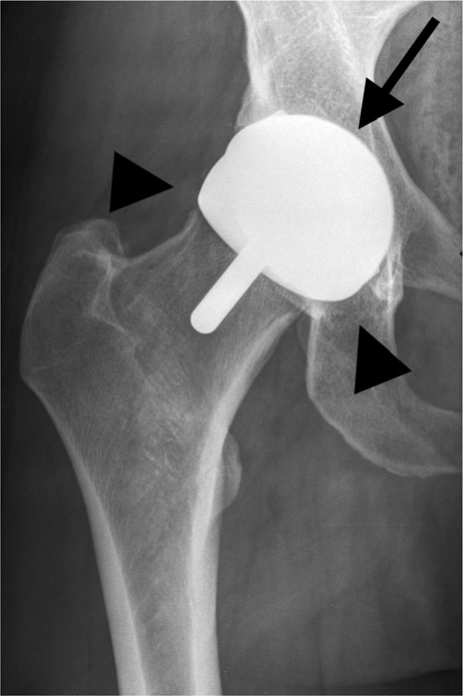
AP radiograph. Cementless resurfacing total hip arthroplasty. In a resurfacing total hip arthroplasty, the femoral head (*arrowheads*) and acetabulum (*arrow*) are replaced. No radiolucent area at the femoral head—acetabulum is noted (metal—on—metal bearing surface)
